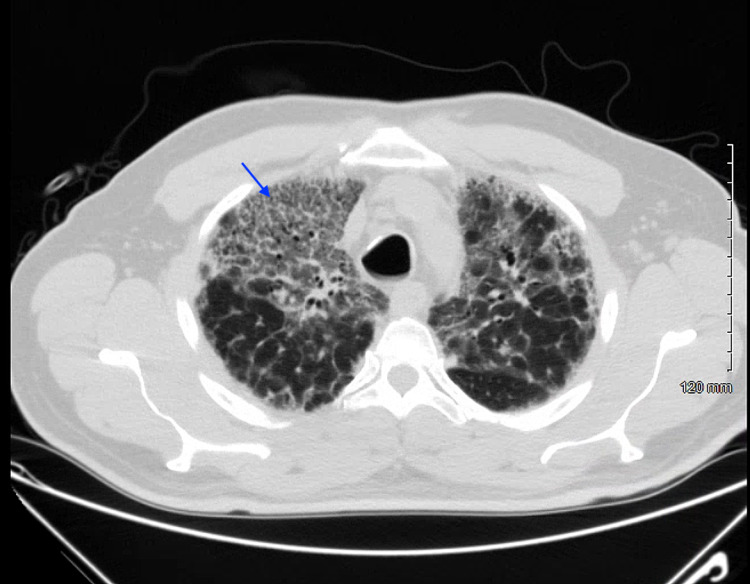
High-resolution computed tomography depicting upper-lobe ground-glass opacities (blue arrow)
